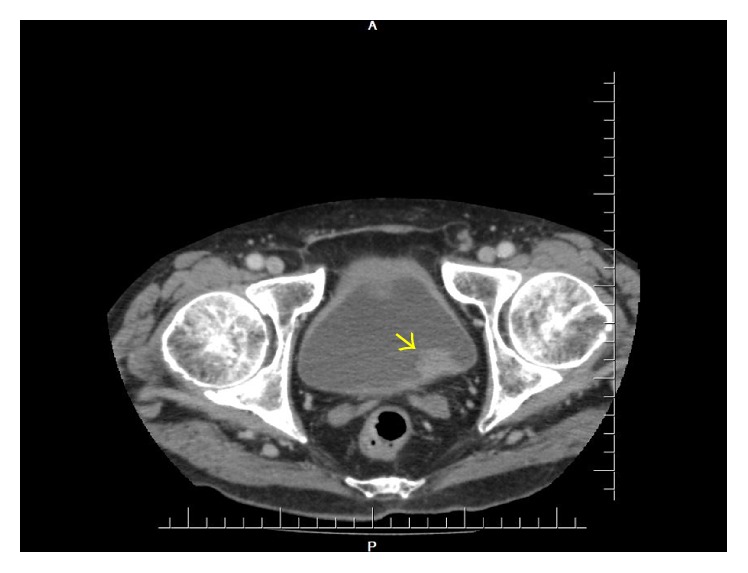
Abdomen CT-scan displaying a vegetation on the posterolateral bladder wall (arrow).
